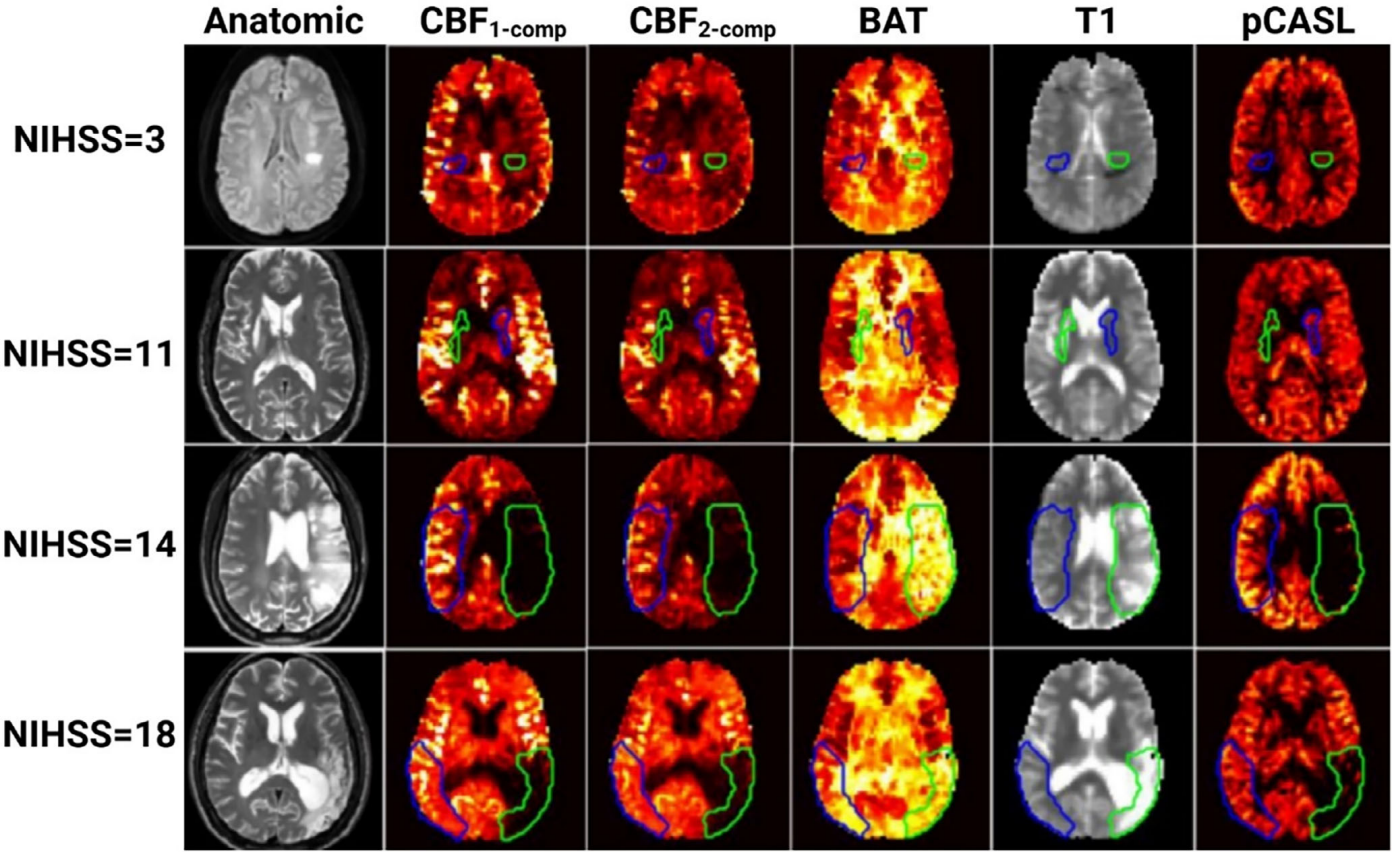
Representative images of MRF‐ASL, traditional pCASL, and anatomical MRI in four stroke patients with NIHSS scores of 3, 11, 14, and 18. Maps include CBF_1_‐comp, CBF_2_‐comp, bolus arrival time (BAT), and T_1_ from MRF‐ASL, with T_1_ representing quantitative measurements (darker signals = longer T_1_). Anatomical images (DWI or T_2_‐weighted) delineate lesions (green) and normal tissue (blue), highlighting perfusion and tissue differences across stroke severities. Adapted from Fan et al. [61], *Stroke*, 2022.
